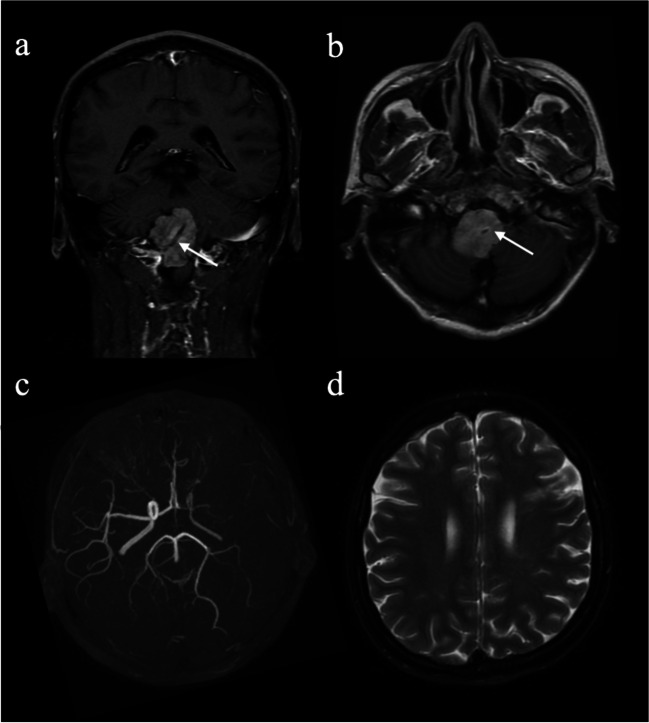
Vascular involvement in ECD patients. a, b Enhanced T1-weighted images show tumoral infiltration around the right vertebral artery (arrows) and compression of the medulla oblongata in a 43-year-old ECD patient. c, d In a 45-year-old man diagnosed to have ECD, MR angiography and T2-weighted image show the vaguely seen left carotid artery and complete occlusion of the left middle cerebral artery and encephalomalacia in the left frontal cortex
